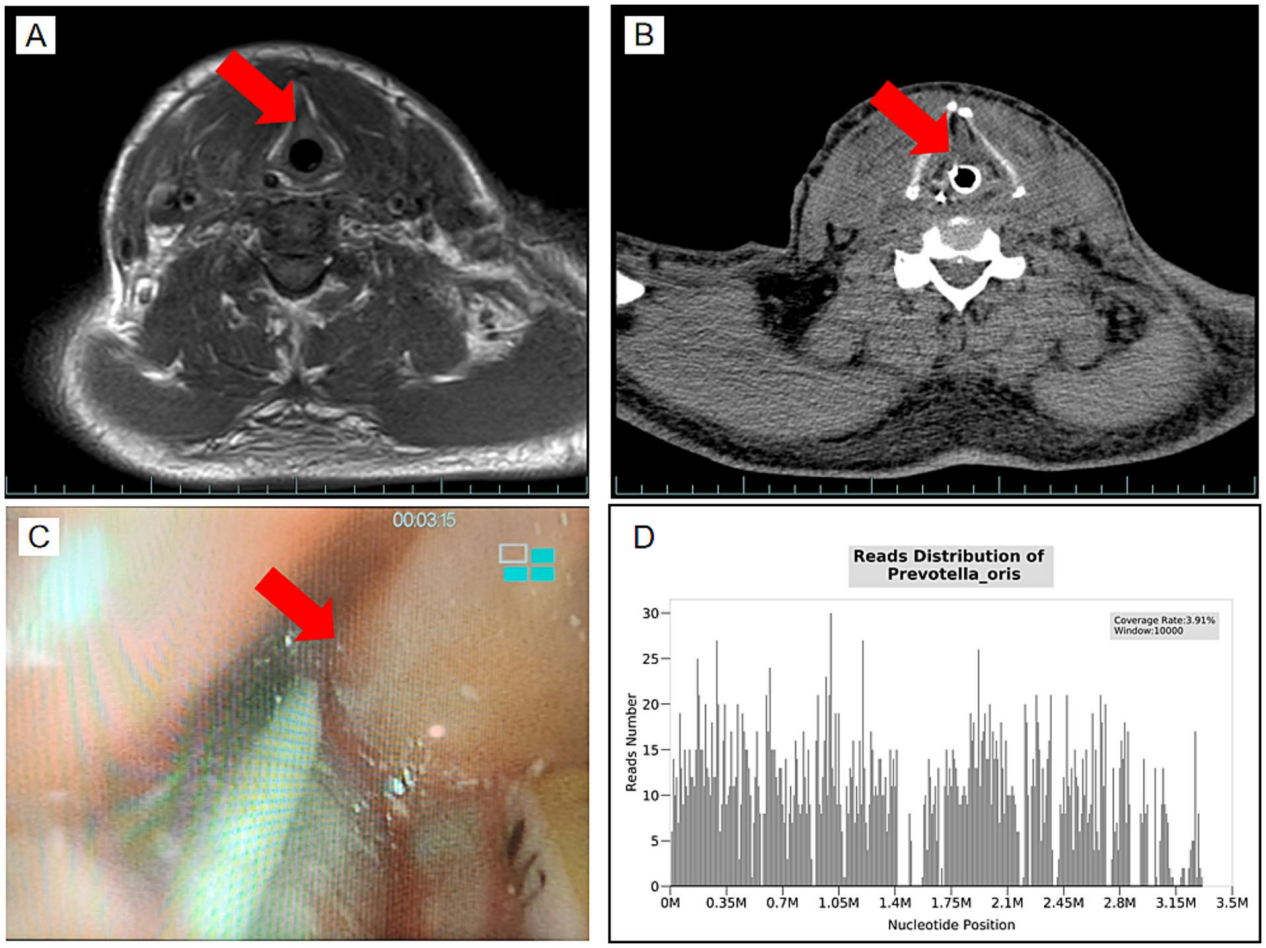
Neck swelling prior to extubation and NGS results. (A) Neck MRI scan showing mild soft tissue swelling in the cervical region (red arrow), indicating localized edema. (B) Neck CT scan showing soft tissue swelling and the presence of inflammation surrounding the airway (red arrow). (C) Laryngoscopy image showing significant edema of the vocal cords and surrounding tissue (red arrow), contributing to difficult extubation. (D) NGS results identifying *Prevotella oris*. *Prevotella oris* was detected by NGS with a coverage rate of 3.91%, indicating its presence among the anaerobic pathogens in the patient’s sample.
